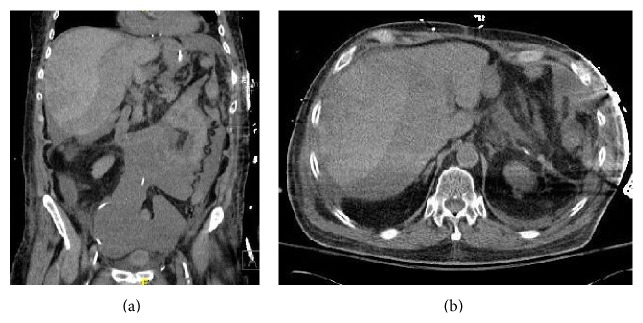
CT abdomen and pelvis. (a) Coronal view and (b) axial view, showing a liver laceration with a large subcapsular hematoma and hemoperitoneum.
